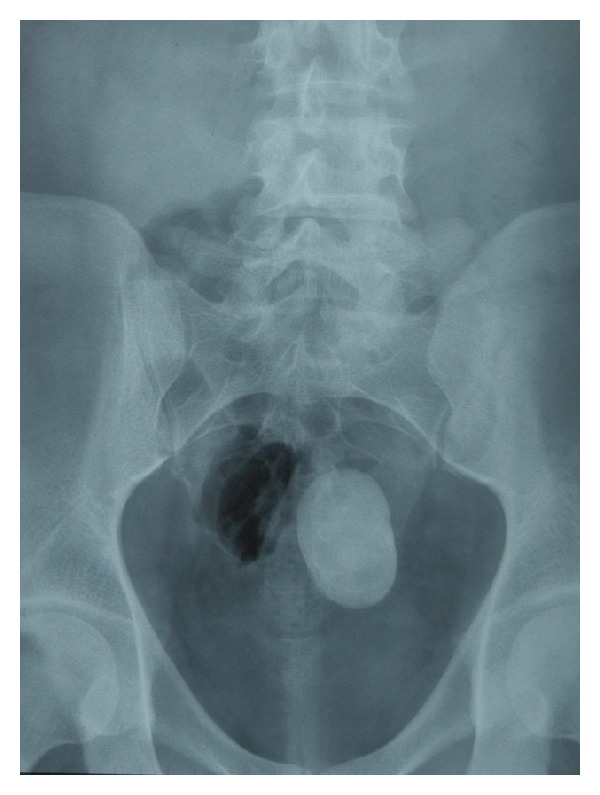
Preoperative direct urinary system radiography.
